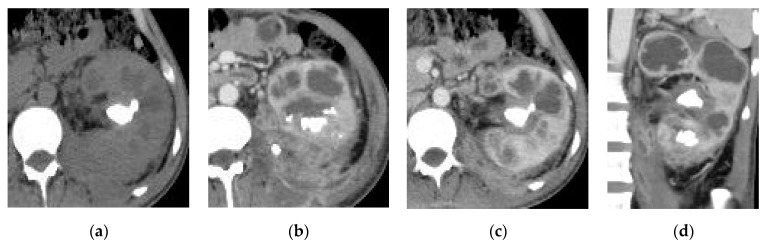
Left xanthogranulomatous pyelonephritis (XGP): (a) axial non-contrast CT image shows an enlarged left kidney with hyperdense stone at the renal pelvis and multiple fluid locules at the renal parenchyma. (b,c) Contrast-enhanced axial and (d) coronal reformatted CT images show multiple stones with multiple cavities at the renal parenchyma and irregular inflammatory lesion at the lower pole of the kidney.
